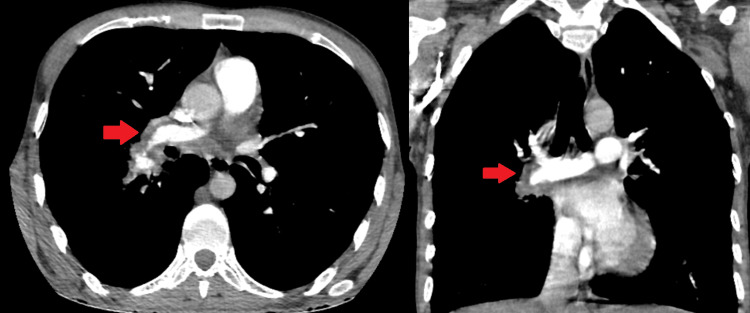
Initial evaluation with CT chest Upon admission, pulmonary thromboembolism and aneurysm in segmentary arteries were observed in the transverse and coronary sections of CT angiography of the thorax CT: computed tomography
